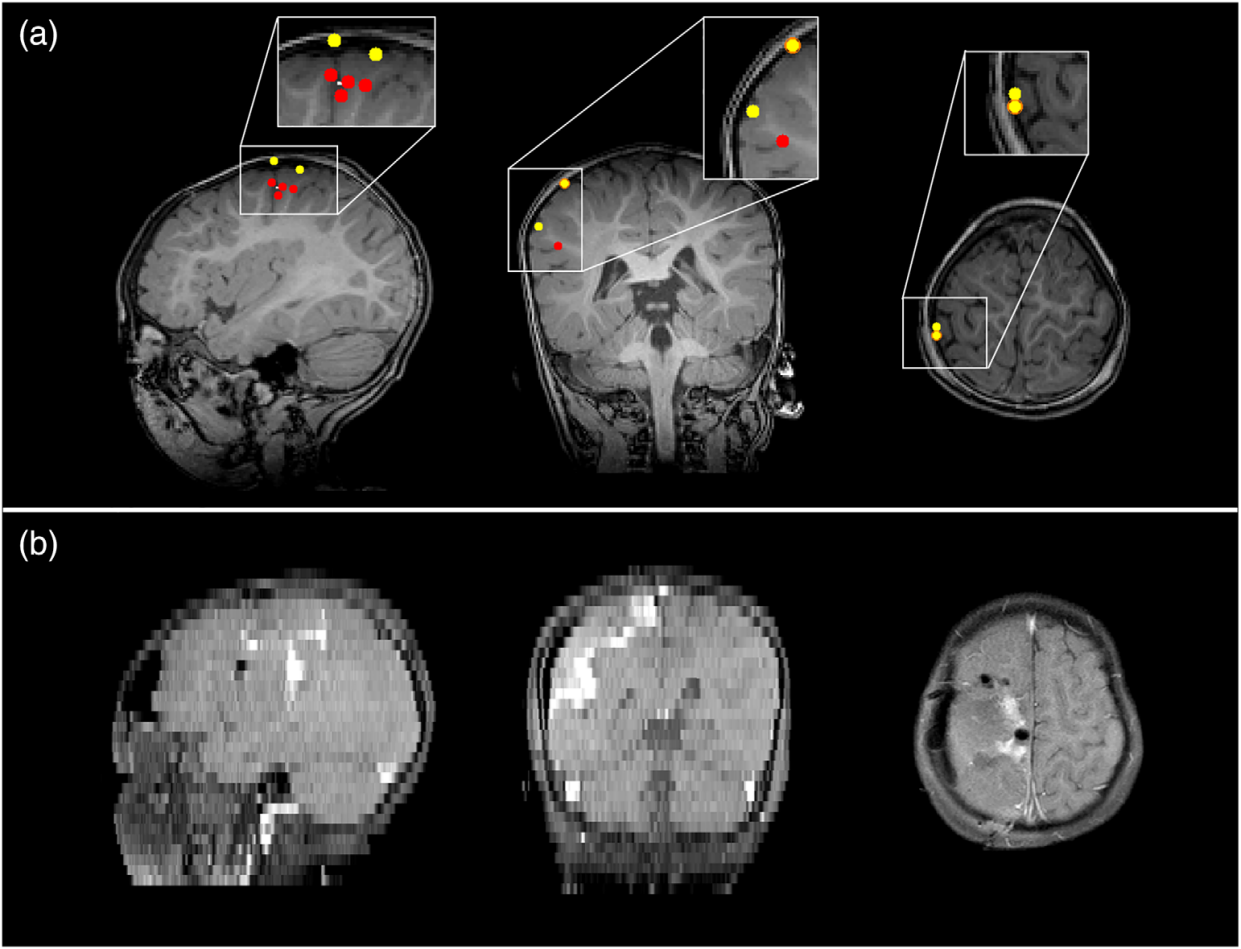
Epilepsy Case 3—magnetoencephalography (MEG) localizations compared with resected area for Patient 8 (multifocal polymorphic). Panel (a) shows The MEG localizations for the EKM method (red markers) and the hidden Markov model method (yellow markers). Panel (b) shows the resected area in the postoperative magnetic resonance imaging (MRI). Note that the postoperative MRI has different resolutions in the sagittal, coronal, and axial planes
